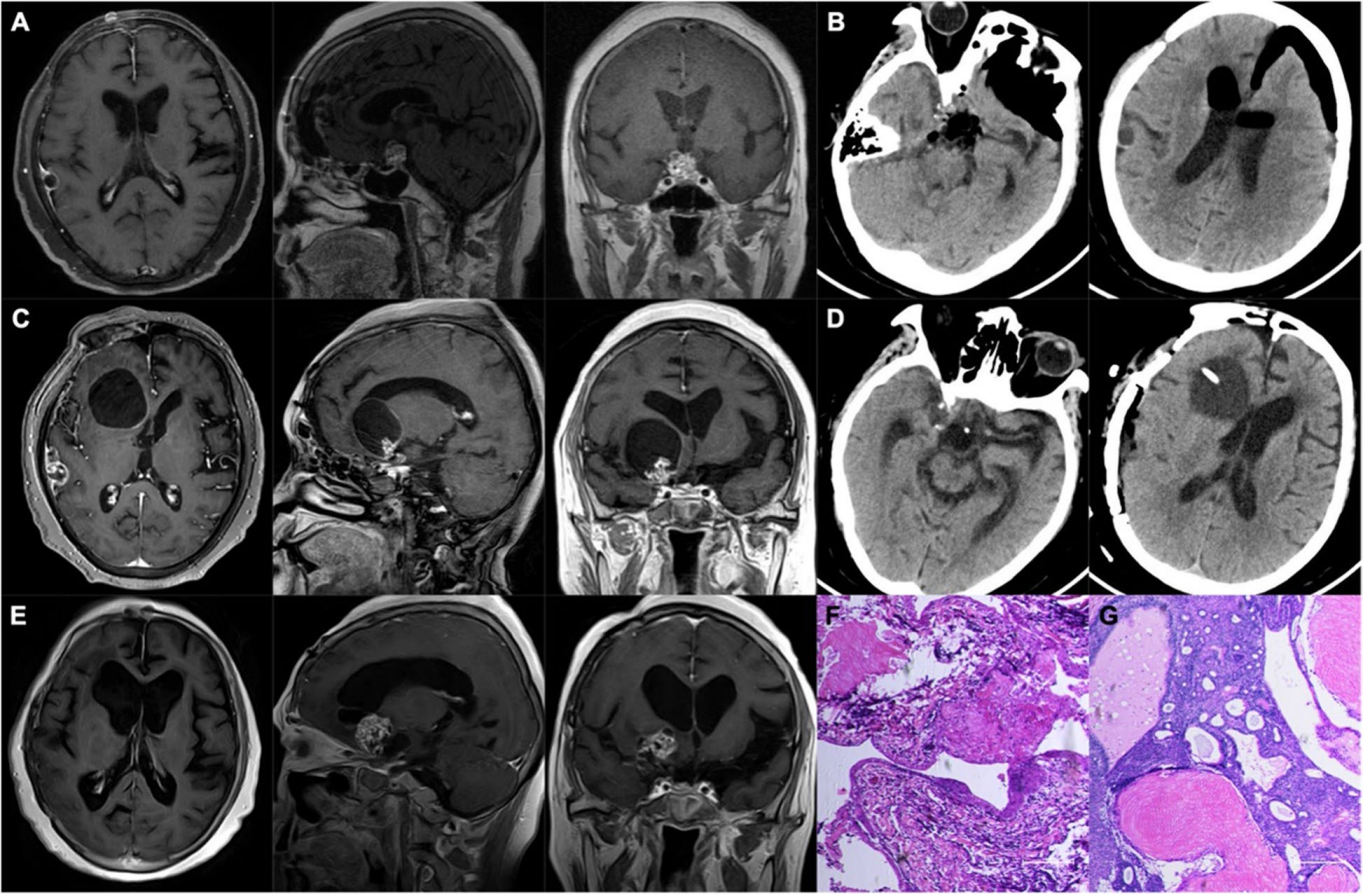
MRI, CT scans, and pathological features of case 2. A Preoperative MRI showed a cystic and solid lesion in the sellar-suprasellar region and a small lesion in the right temporal lobe in 2019. B CT scan after endoscopic transsphenoidal surgery. C MRI scan showed tumor recurrence with cyst in the suprasellar region and enlargement of the lesion in the right temporal lobe in 2020. D CT scan after transcranial surgery and Ommaya reservoir implantation. E MRI scan showed tumor recurrence again in the suprasellar region in 2021. F The pathological features of suprasellar lesion showed adamantinomatous craniopharyngioma. G The pathological features of right temporal lobe lesion also showed adamantinomatous craniopharyngioma
